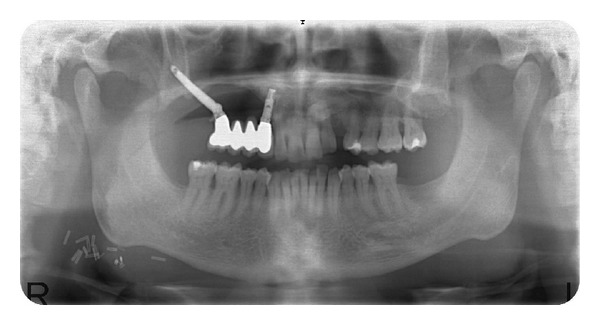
Radiographic view after 1 year follow-up.
